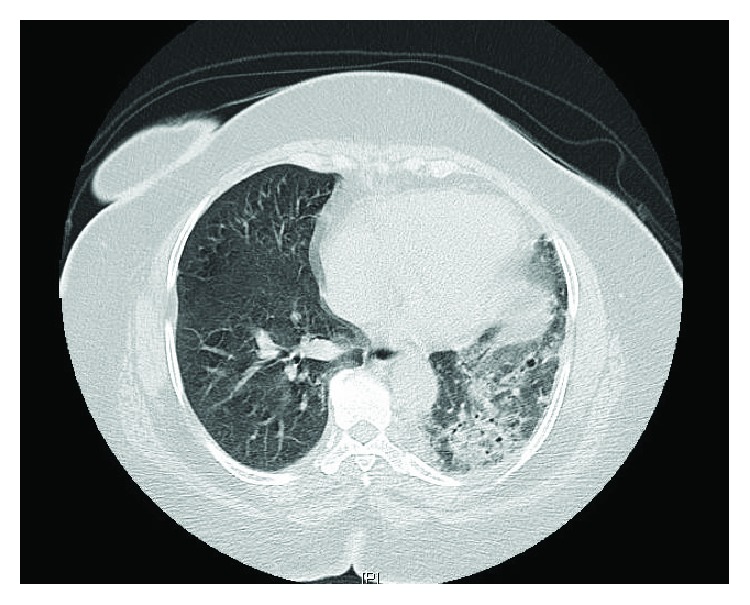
Axial CT of the chest with consolidative changes to the left lung and hypertrophied bronchial arteries.
